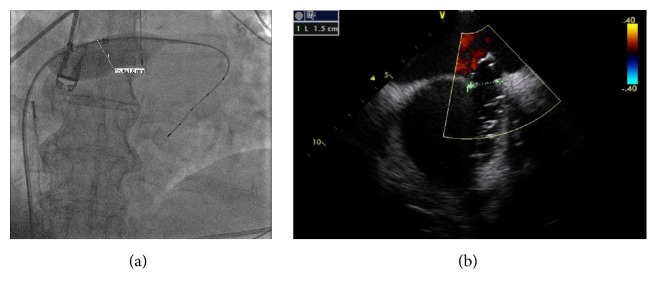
(a) Sizing balloon showing the diameter of the ASD. (b) Intracardiac echocardiogram showing the diameter of the ASD.
